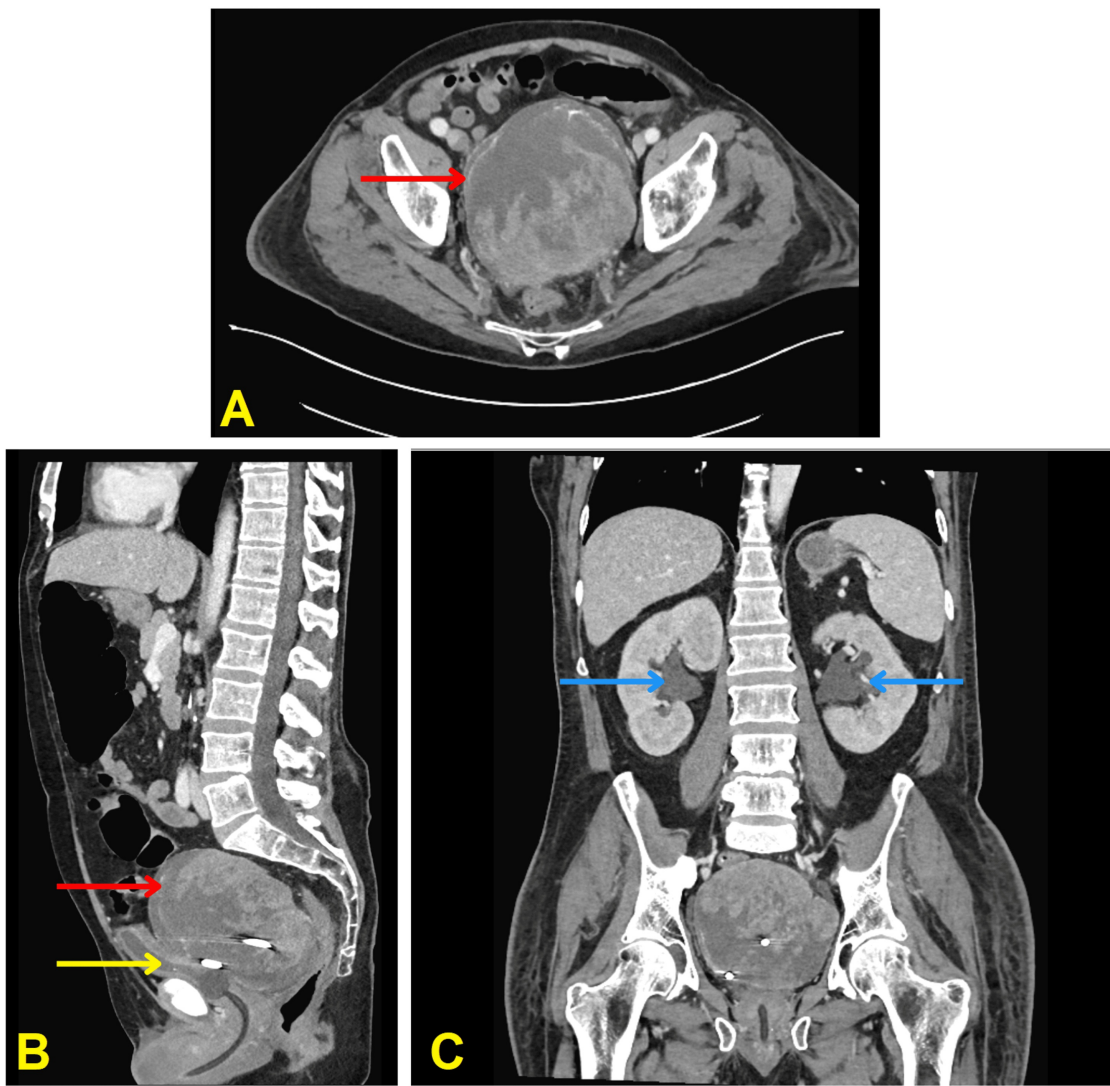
CT scan of abdomen and pelvis with contrast (A) axial view, (B) sagittal view, and (C) coronal view. The urinary bladder is collapsed (yellow arrow in panel B) with a Foley catheter in place. Complex fluid collection corresponding with internal density/mass lesion, with an anterior approach suprapubic drainage catheter. The catheter terminates in the complex fluid collection which measures 12.7 cm AP by 11.4 cm transverse by 10.4 cm craniocaudal (red arrow in panels A, B). Bilateral pelviectasis and hydroureter (blue arrows in panel C) CT: computed tomography
